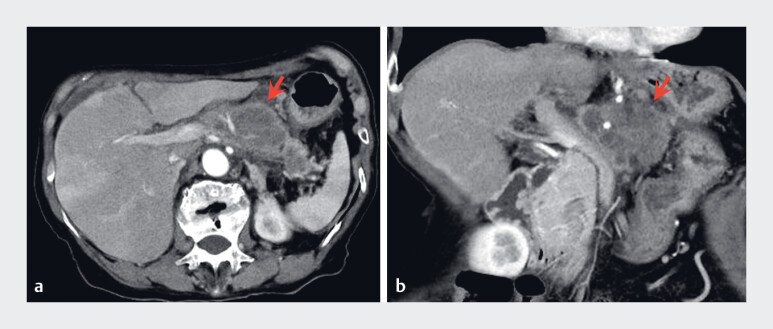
Computed tomography images of the 83-mm mass in the pancreatic body (arrows).
